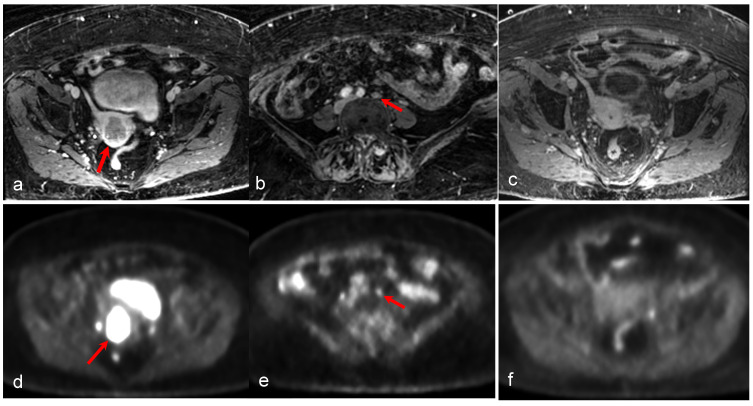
An 81-year-old woman with high-grade serous carcinoma of an endometrial origin. FDG PET/MRI was obtained for staging. Axial-focused PET image (d) demonstrating focal intense FDG uptake within the lower uterus and upper cervix, corresponding to a hypoenhancing mass seen on the axial T1-weighted post-contrast image (a). Additionally, an 8 mm short axis left common iliac chain node with mild FDG uptake was noted (b,e). There was no evidence of more distant metastatic disease. The patient was treated with pelvic radiation including boost to the left iliac chain lymph node, and subsequent chemotherapy. Follow-up axial T1-weighted image (c) and PET/MR (f) revealing complete metabolic response with absent FDG uptake within the mass, and complete resolution of abnormal enhancement with only a small amount of non-enhancing fluid in the endometrial canal. (Courtesy of Eric C. Ehman, MD, Department of Radiology, Mayo Clinic, Rochester, MN, USA).
